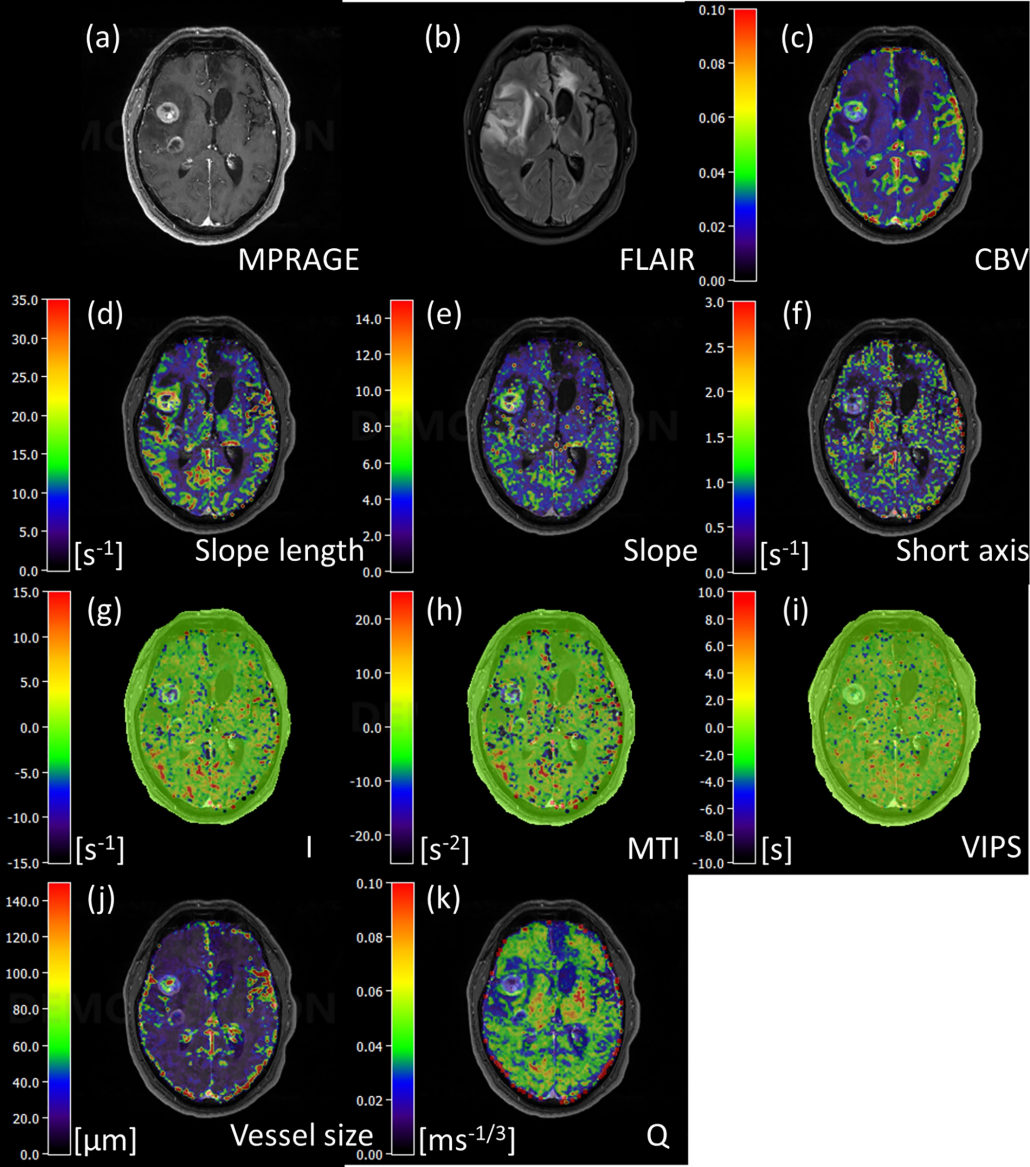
(a) Contrast-enhanced T1-weighted MPRAGE image. (b) FLAIR image. (c) rCBV. (d) Slope length. (e) Short axis. (f) Slope. (g) Distance map. (h) MTI. (i) VIPS. (j) Vessel size. (k) Q map.
